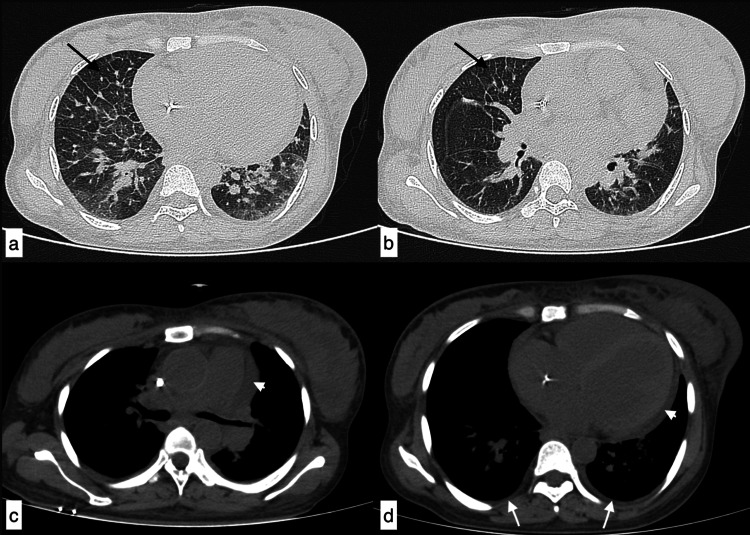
Pulmonary oedema A 25-year-old female patient with SLE presented with shortness of breath and orthopnoea of three-day duration. Axial HRCT chest images in the lung window (a, b) revealed smooth interlobular interstitial septal thickening in both lung fields with diffuse ground-glass opacities in both lung fields, giving a crazy paving appearance (black arrow). Additionally, cardiomegaly with mild pericardial effusion (arrowheads) and minimal bilateral pleural effusions (arrows) were observed in mediastinal window images (c, d). Findings were consistent with pulmonary oedema. SLE: systemic lupus erythematosus; HRCT: high-resolution CT
